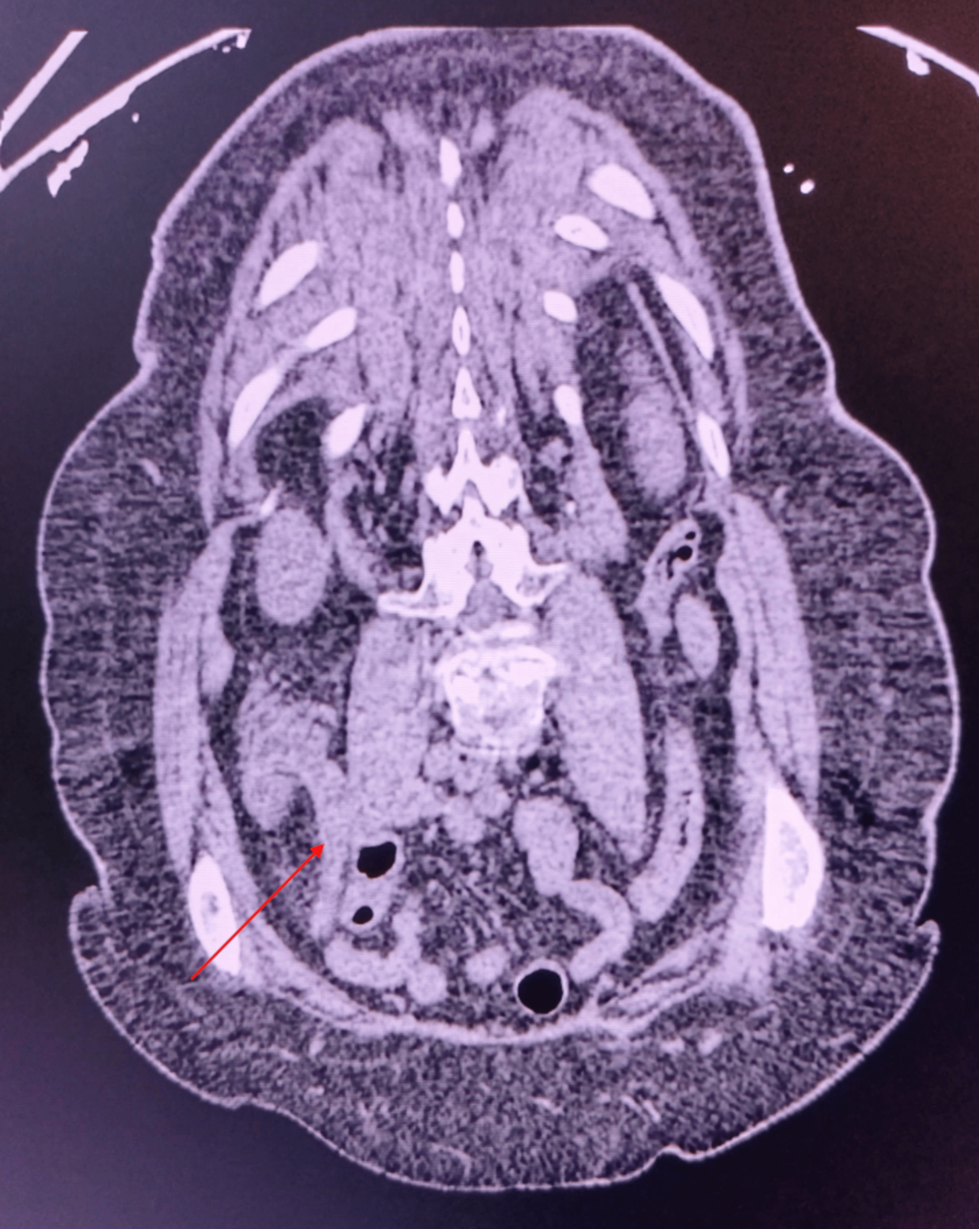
Abdominopelvic CT Case 3. Red arrow showing the inflamed appendix with slight densification of the adjacent adipose planes, without signs of diverticulitis.
